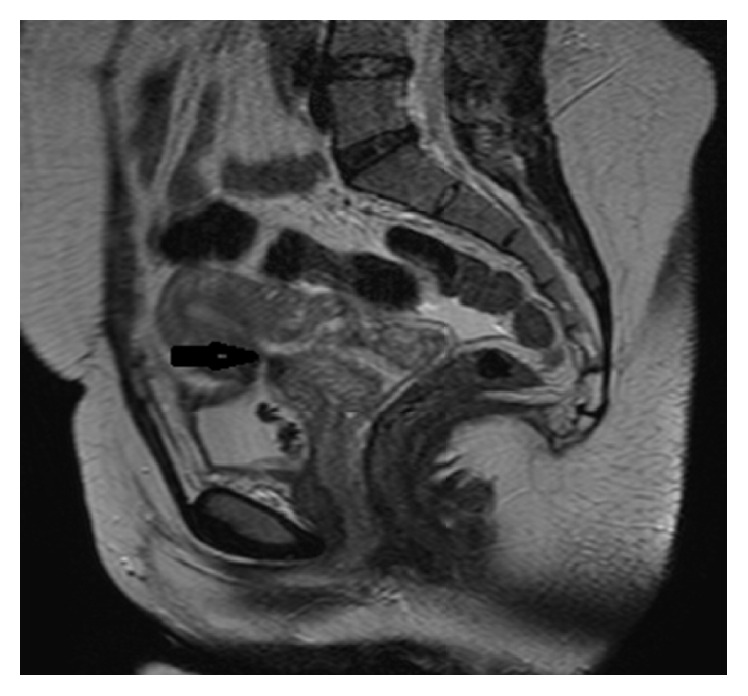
Fistula tract between the uterus anterior wall and bladder posterior wall in MRI.
